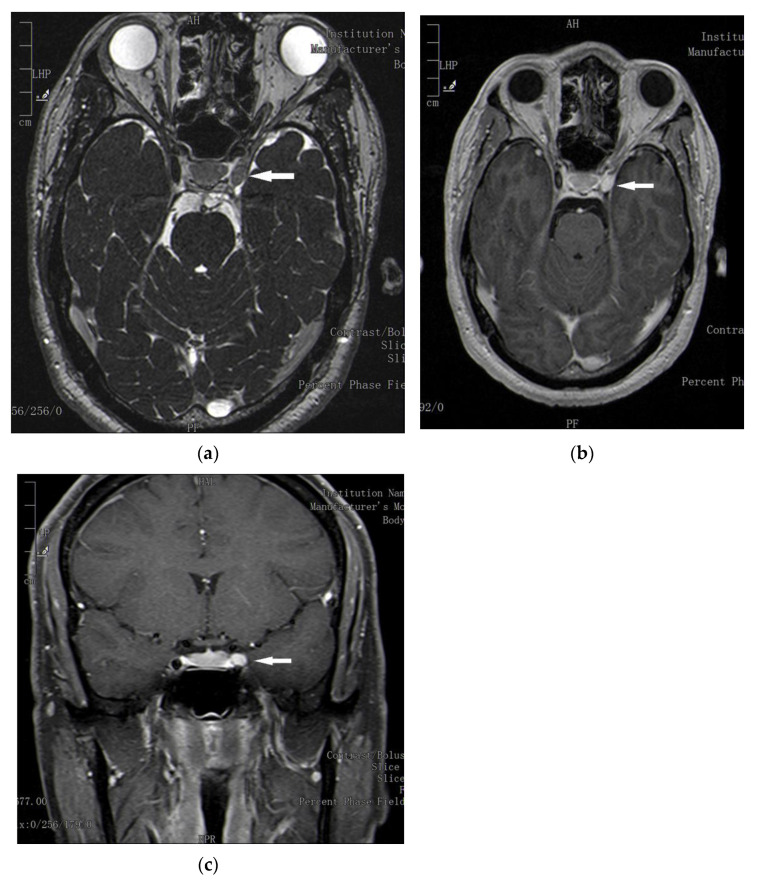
Brain magnetic resonance imaging demonstrating (see respectively white arrow) a possible oculomotor nerve schwannoma in the left sinus cavernous, highly enhanced by gadolinium (Gad). (a) T2-weighted axial magnetic resonance imaging (MRI) brain without Gad. (b) T1-weighted axial brain MRI with Gad. (c) T1-weighted coronal brain MRI with Gad.
